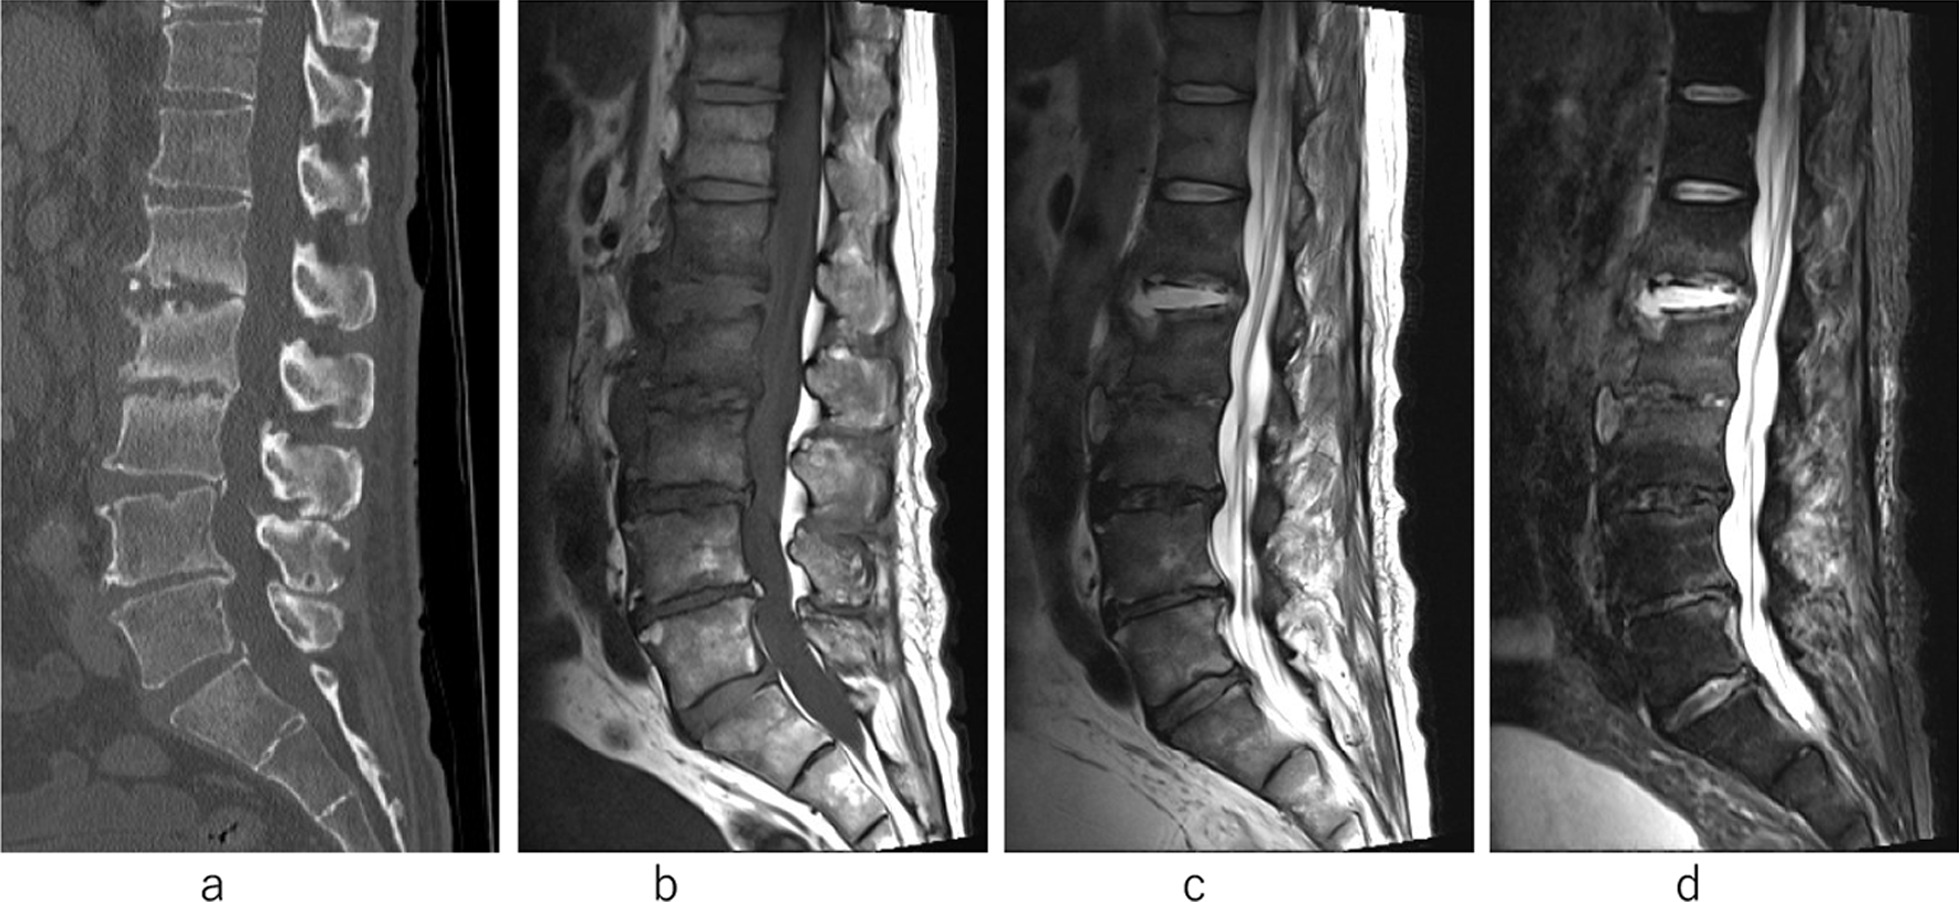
At the second admission. There was slight endplate irregularity in the L1–2 and L2–3 disc spaces on computed tomography (a). There is fluid signal in the L1–2 intervertebral space, which was low intensity on T1-weighted magnetic resonance images and high intensity on T2-weighted MR images and short tau image recovery in MRI (b–d)
